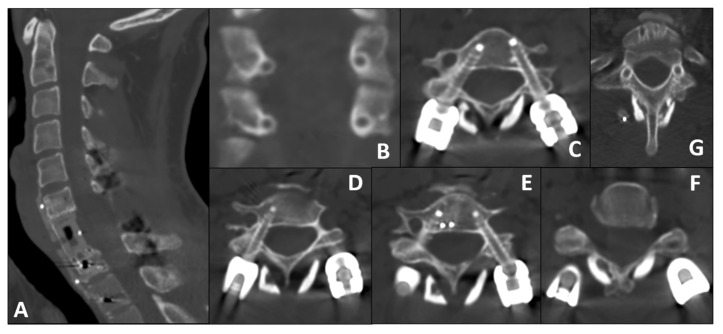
Three-month postoperative CT scan (bone window) confirming stable circumferential reconstruction (A) Midline sagittal view showing adequate press-fit of the anterior cage without subsidence. (B) Coronal reconstruction at C5–C6 demonstrating the planned medial trajectory of the right-sided pedicle screws, unchanged compared with the immediate postoperative CT and without signs of loosening. (C) Axial view at C5 confirming correct screw positioning and construct stability. (D,E) Axial views showing the right and left pedicle screws, respectively, with no evidence of mobilization; the medial cortical encroachment on the right side is unchanged. (F,G) Axial views at the cranial and caudal ends of the posterior construct demonstrating early osseous integration of the structural bone graft. No radiological evidence of residual or recurrent disease is observed.
